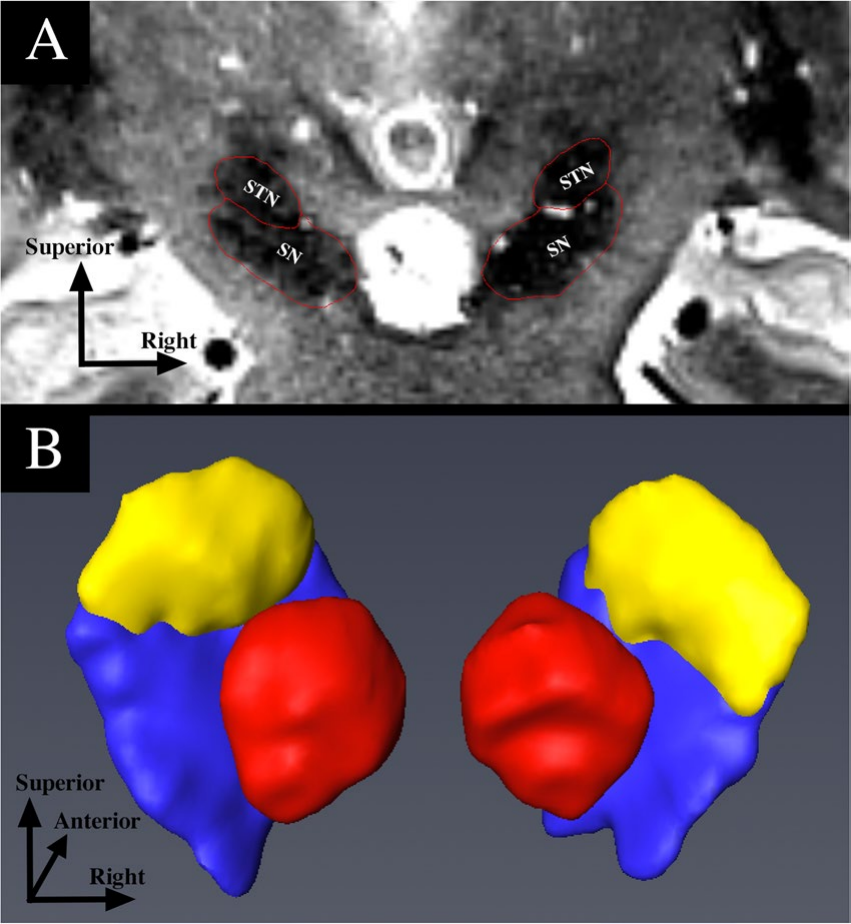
Example of STN segmentation and 3D reconstruction for one patient in the coronal orientation. (A) Identification of the STN on a T2 MRI slice. (B) 3D rendering of the STN, SN and RN. Yellow = STN. Blue = SN. Red = RN. STN = subthalamic nucleus. SN = substantia nigra. RN = red nucleus.
